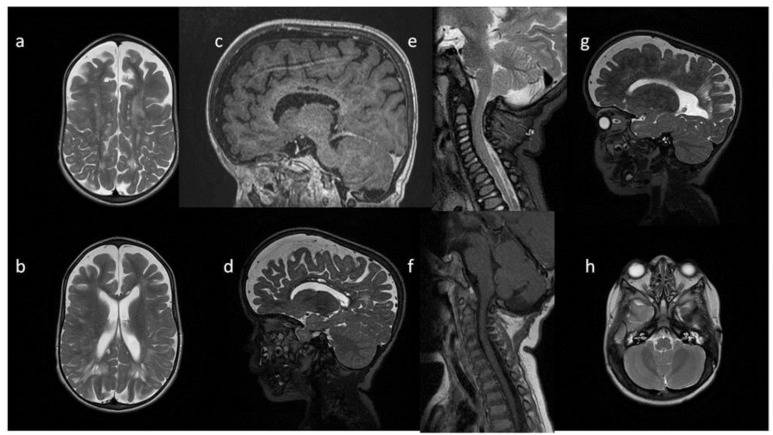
Brain MRI. Patient 4, 1st exam. (a–d) axial T1 & T2; (e–h) sagittal T1, T2, Flair T2, T1. Findings: (a,c,g) enlarged periventricular spaces with characteristic location in corpus callosum; (a,c,g) abnormal white matter signals; (b) posterior fossa horns, mastoid cells fluid; (d) optic nerve sheath enlargement, ethmoid sinus fluid; (e,f,h) J-shaped sella turcica, dysplastic dens; (e,g) skull deformation.
